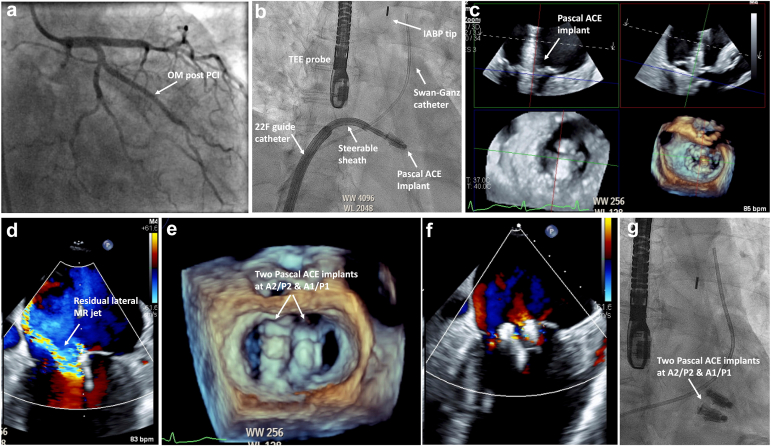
Intraprocedural imaging (a) Post-PCI. (b) LA access and Pascal ACE positioning. (c) Multiplanar reconstruction for TEE-guided implant positioning and leaflet capture. (d) Residual MR post A2/P2 implant. (e) 3D TEE post deployment of second implant. (f) Completion shot with 2 implants in appropriate position and minimal trace MR. (g) Completion fluoroscopic image. Abberviations: IABP, intra-aortic balloon pump; LA, left atrium; MR, mitral regurgitation; OM, obtuse marginal; TEE, transesophageal echocardiogram.
